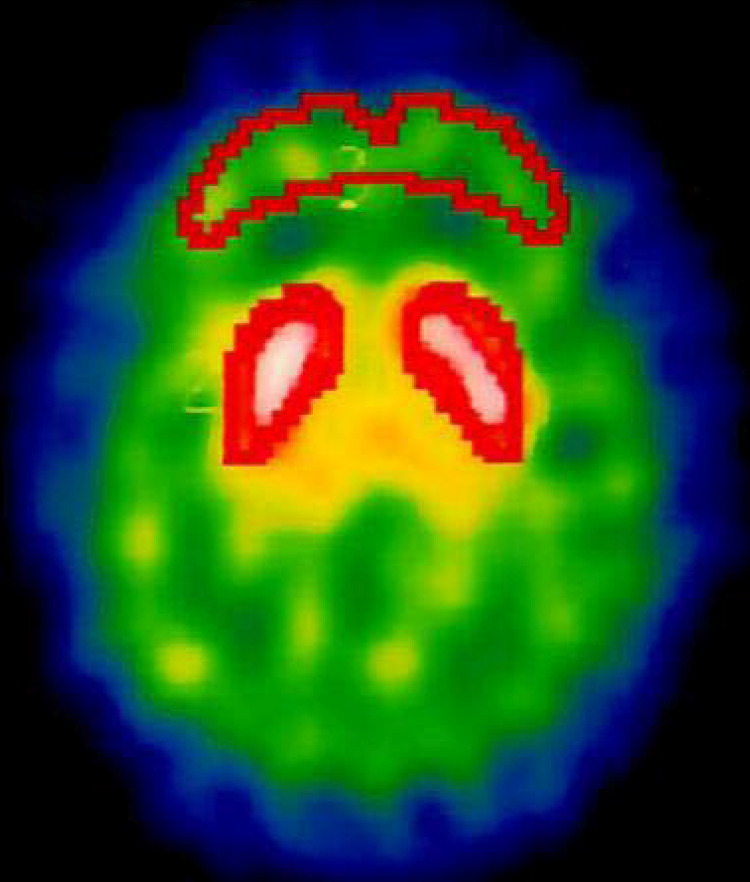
The regions of interest (ROIs) were placed over the striatum and the frontal cortex. The ROIs were drawn directly on the SPECT images by an experienced nuclear-medicine physician who was blind to the participants' clinical status and data.
